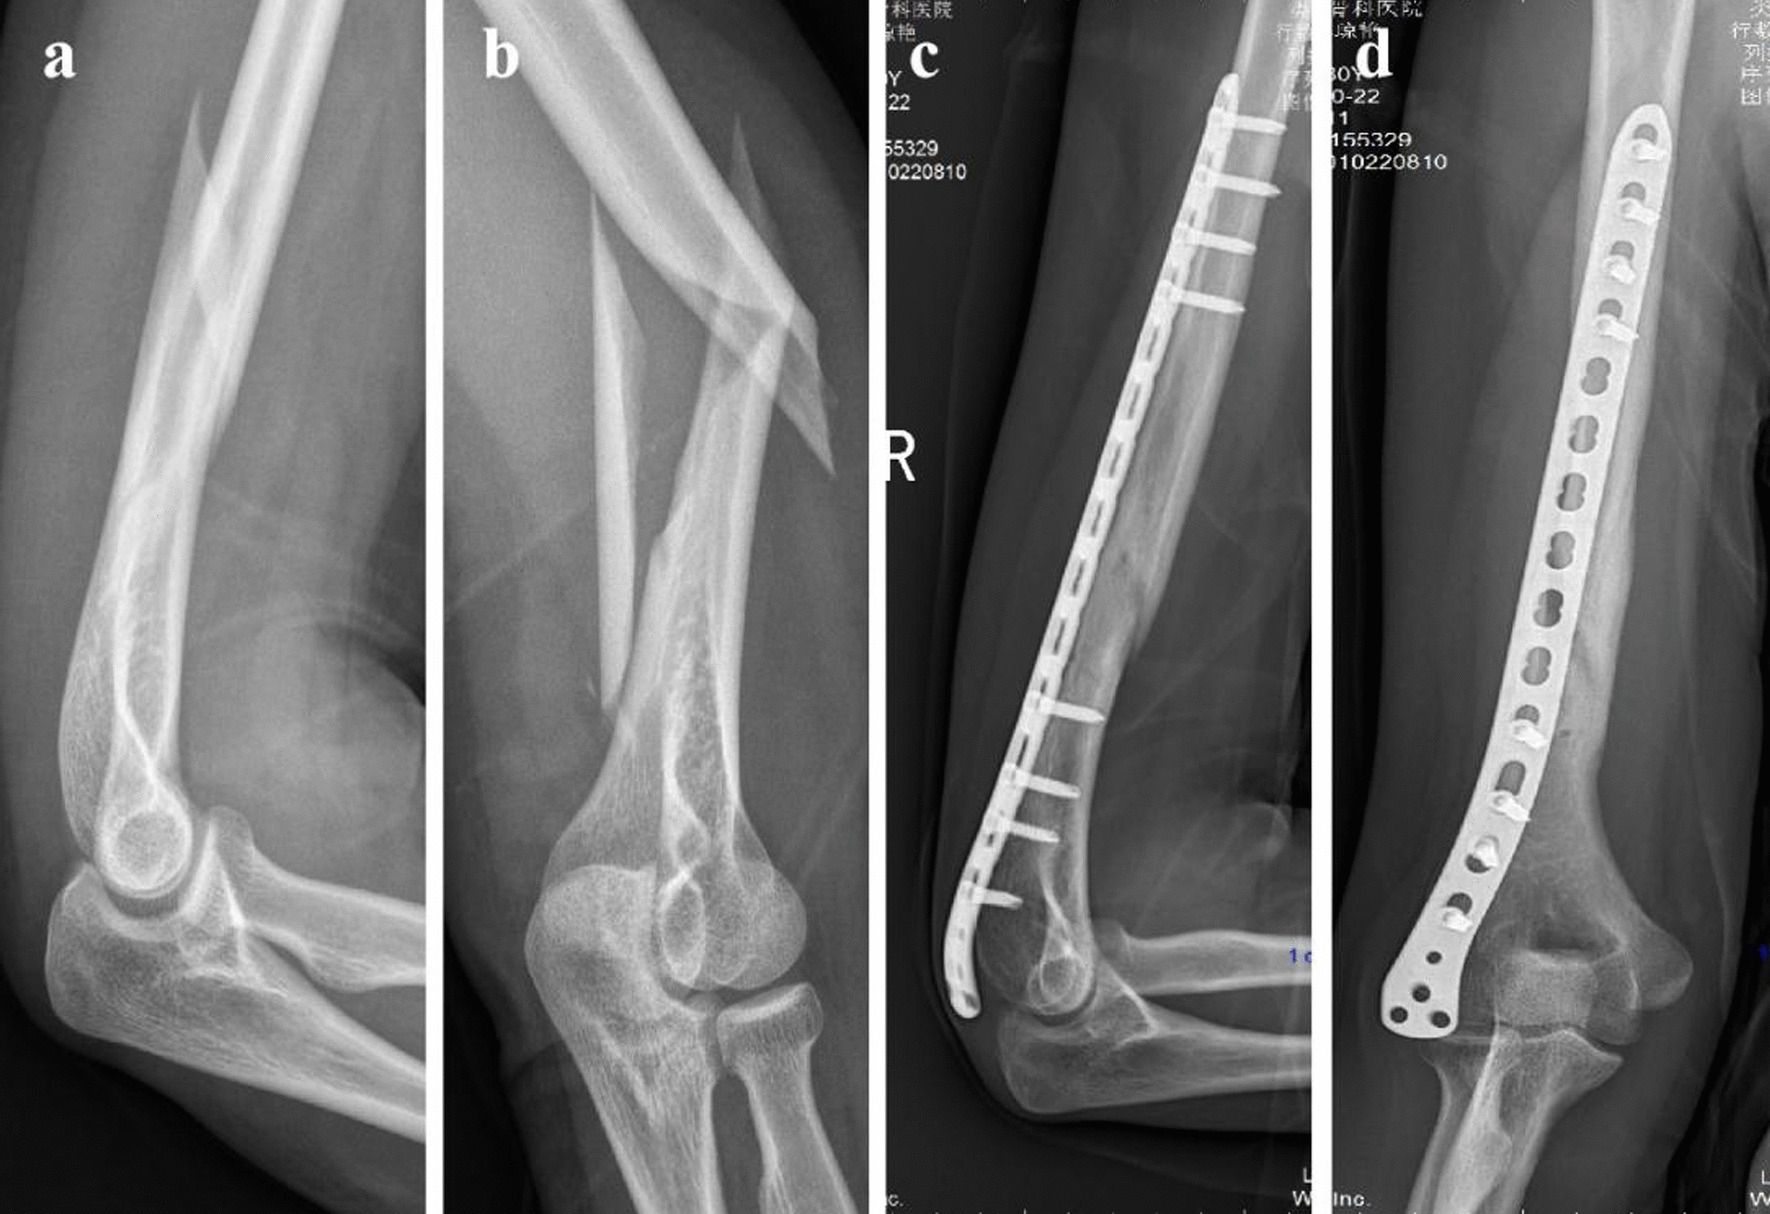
The MIPPO used in Case 1: a 31-year-old female, indirect violence in a fall leads to the right middle-inferior humerus fractures. a Preoperative lateral X-ray radiograph of the middle-inferior humerus fractures. b Preoperative anteroposterior X-ray radiograph of the middle-inferior humerus fractures. c The lateral X-ray radiograph of full-length humerus 8 months after MIPPO. d The anteroposterior X-ray radiograph of the full-length humerus 8 months after MIPPO
